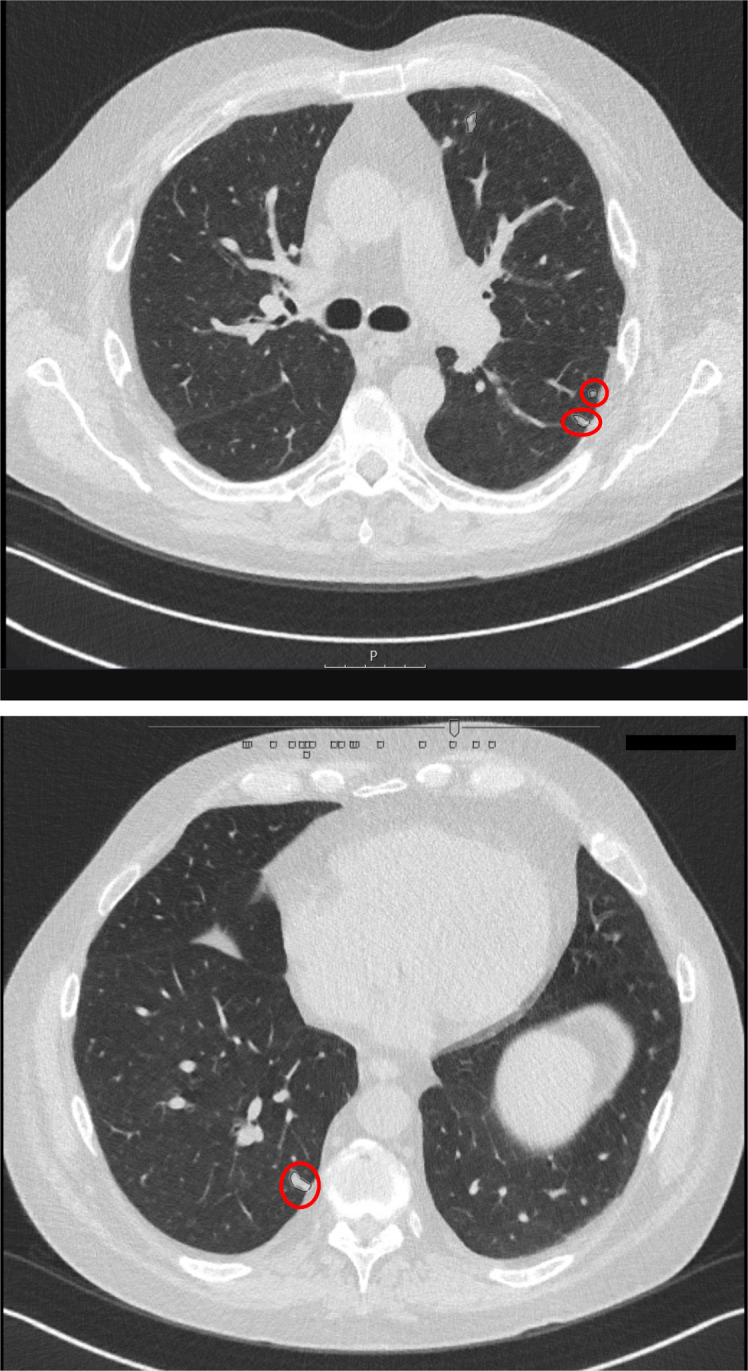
HRCT pulmonary imaging. Two representative stills from the HRCT pulmonary imaging obtained in this patient. The red circles mark intrapulmonary nodular opacities, suggestive of pulmonary cryptococcal disease. HRCT, high-resolution CT.
